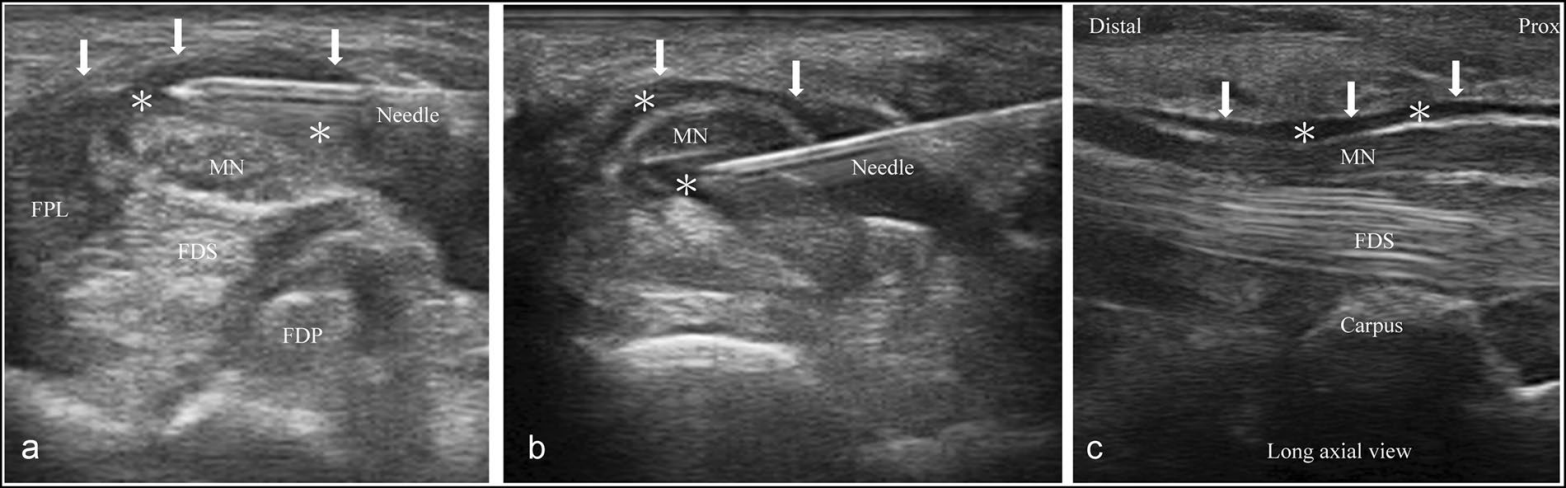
Ultrasonographic imaging. (a) Transverse view showing the median nerve (MN) peeled off from the flexor retinaculum (arrows) using 2 mL of PRP via hydrodissection (asterisk). (b) Transverse view showing the MN separated from the inferior underlying subsynovial connective tissue using an additional 1 mL of PRP via hydrodissection (asterisk). (c) Long axial view showing the distribution of PRP throughout the proximal-to-distal area of the carpal tunnel (asterisk). MN: median nerve; FPL: flexor pollicis longus; FDS: flexor digitorum superficialis; FDP: flexor digitorum profundus.
